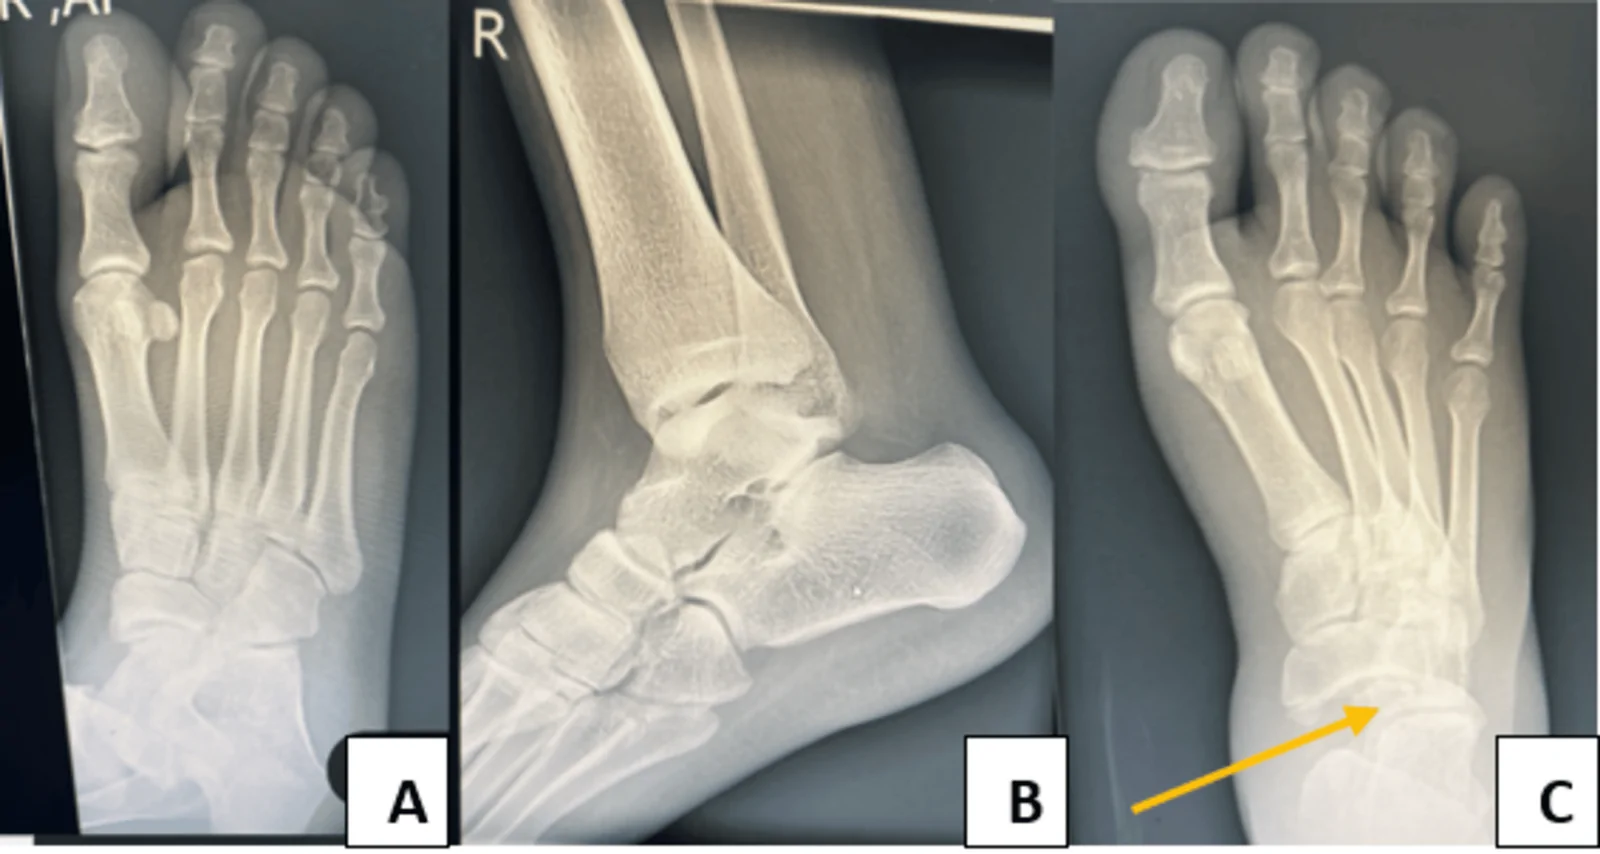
Preoperative radiographs taken three weeks after injury (A) Anteroposterior view, (B) lateral view, and (C) oblique view (arrow indicating right foot medial swivel injury)
